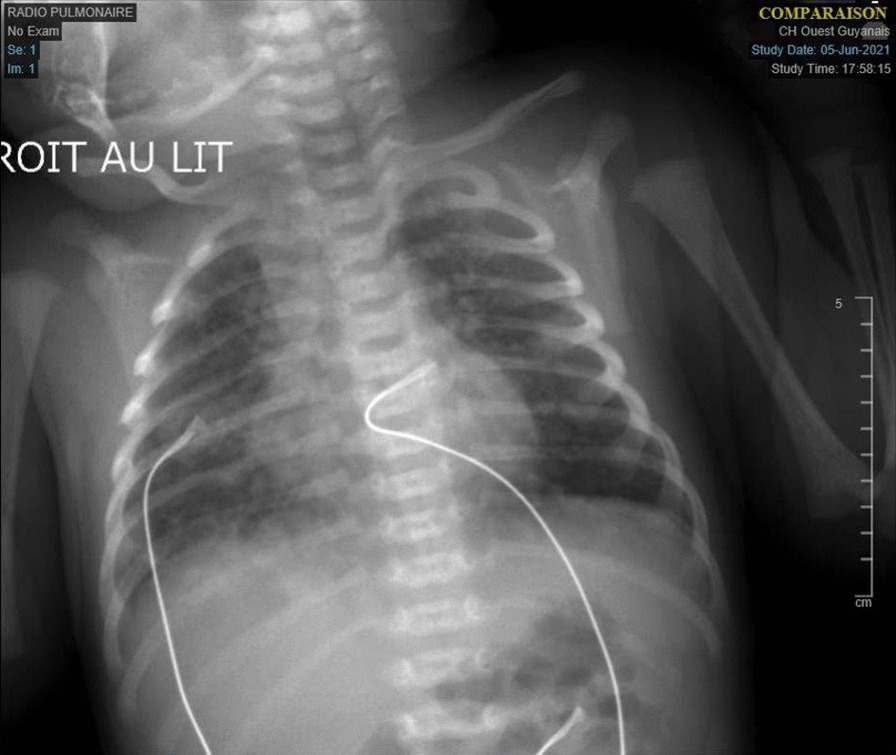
Chest radiography showed bilateral opacities
